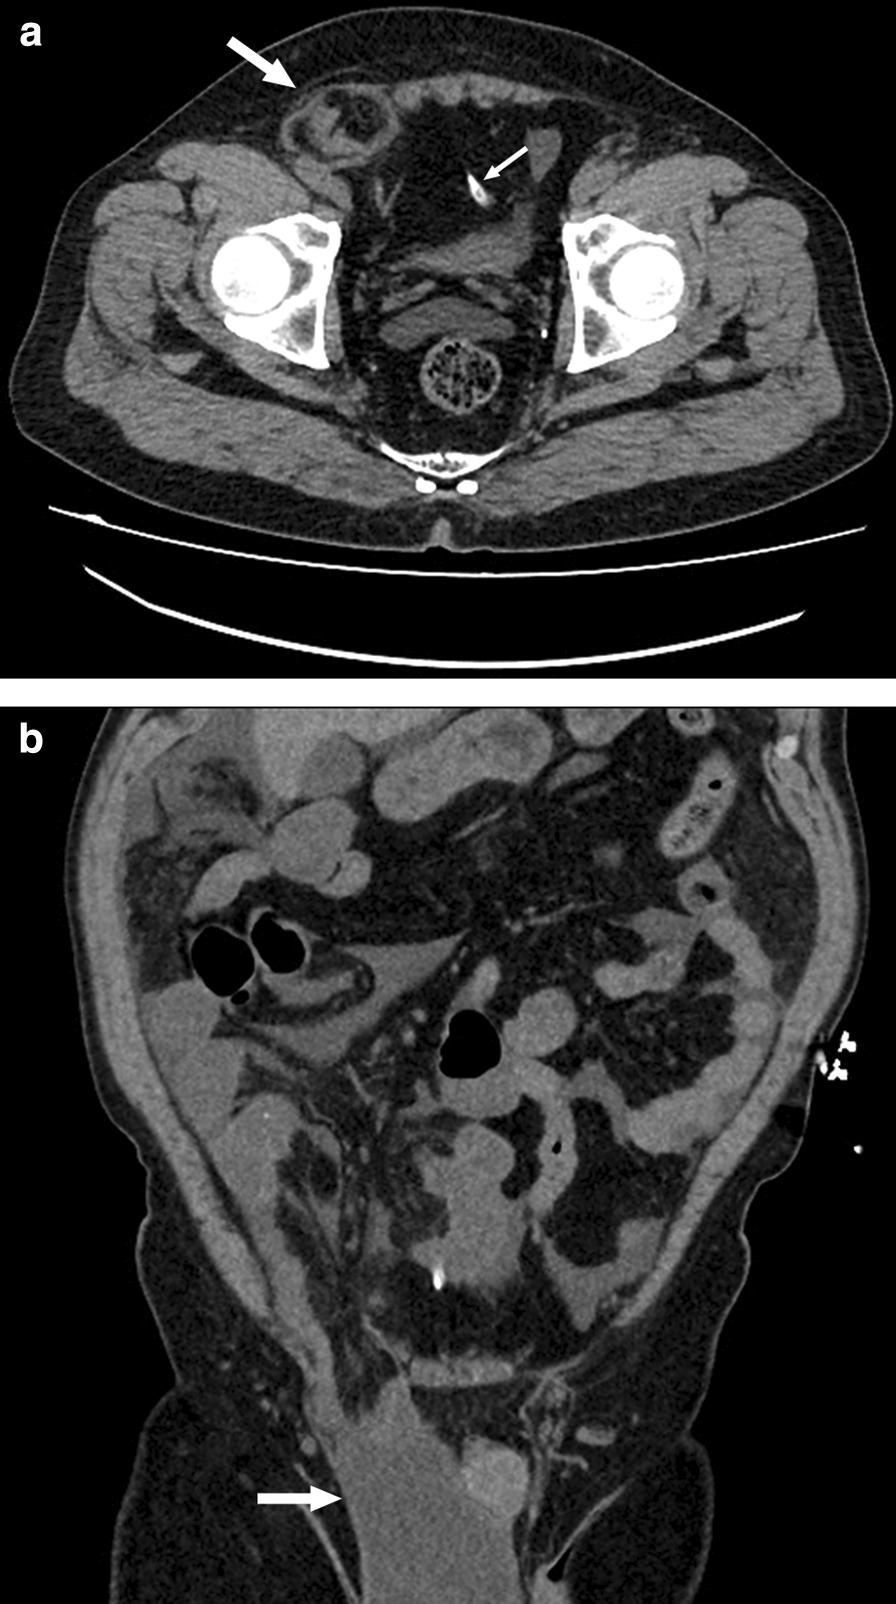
Nonenhanced CT images of patients with CAPD and hernia. a Axial CT image show a right inguinal hernia (thick arrow) and a CAPD catheter (thin arrow). b Coronal CT image show right inguinal hernia with dialysate (arrow)
